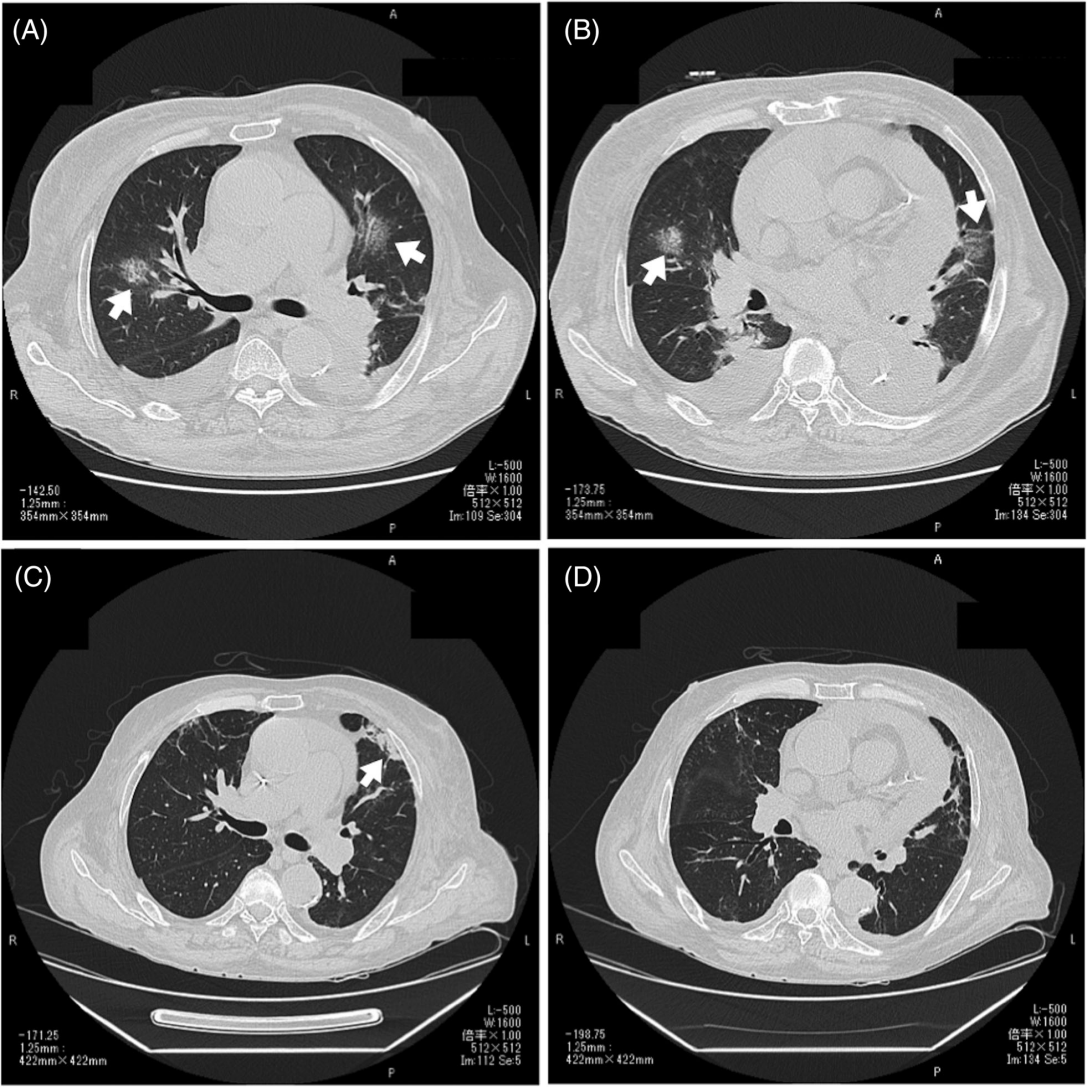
Postoperative day 3 chest computed tomography (CT) (A, B) showed ground‐glass‐like patchy shadows (white arrows), pericardial fluid and bilateral pleural effusion in both lungs. On postoperative day 28 chest CT (C, D), pleural effusion was decreased compared to the onset of COVID‐19 infection, with only mild post‐pneumonia fibrosis (white arrow) observed in the upper left lobe
